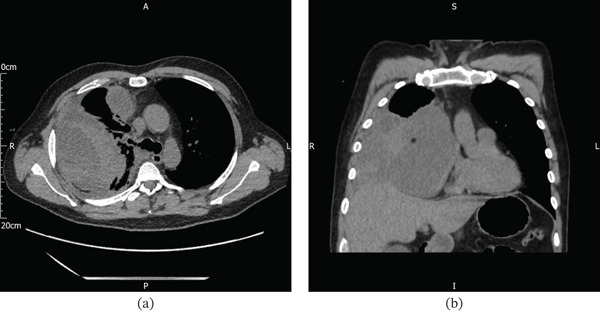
(a and b) Thoracic CT showing hyperdense pleural‐based lesions, with fluid and heterogeneous soft tissue.
